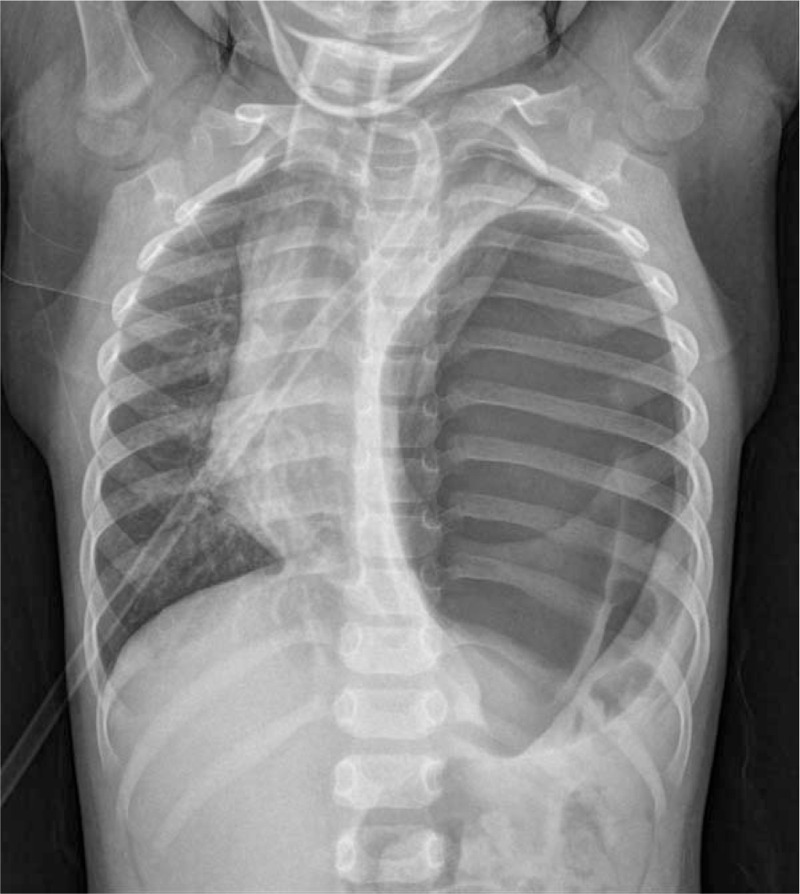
Chest x-ray showing air shadowing filling the entire left thorax and mediastinum shifting.
